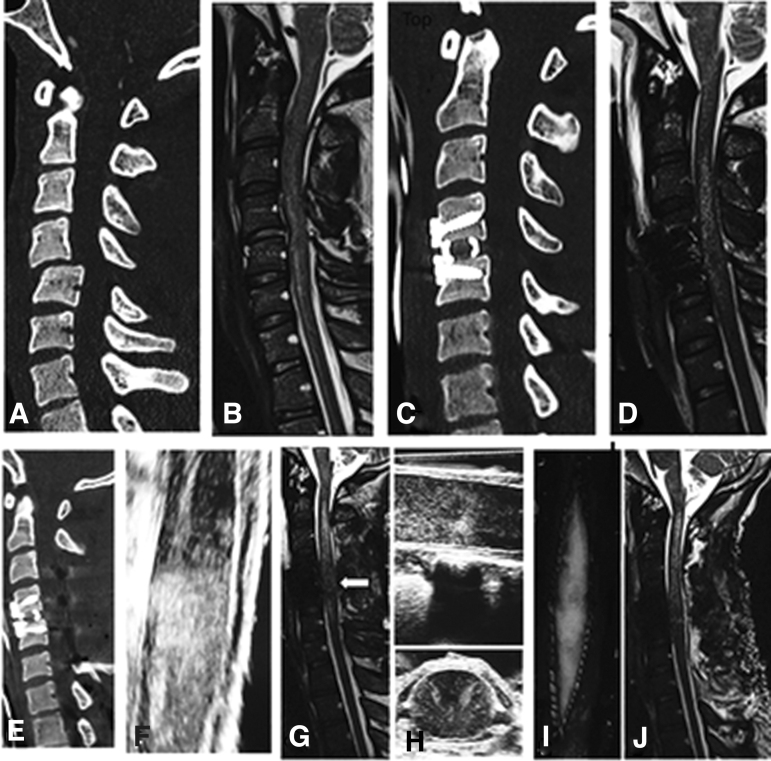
Midsagittal computed tomography and magnetic resonance imaging (MRI) views (plates A and B) from a 20-year-old male patient with C4/5 unilateral locked facets and quadriplegia during a friendly game of wrestling. Plate A indicates translation rotation injury between C4 and C5 (AOSpine injury type C^28^) vertebral bodies 6 h after trauma. Nine hours after traction reduction, the MRI indicated lack of cerebrospinal fluid (CSF) interface in front and in the back of the spinal cord from C2–C6 and an intramedullary lesion length of 73.1 mm. (plate B). After anterior cervical discectomy and fusion, MRI (plate D) indicated continued absence of CSF in front and in the back of the spinal cord. The patient had a second stage surgery that was laminectomy. Real-time intraoperative ultrasonography after laminectomy revealed spinal cord swelling with a thin interface in front of the spinal cord; however, the surgeon was not convinced expansive duraplasty was necessary (plates E and F). Post-operative MRI (plate G) revealed absence of CSF around the spinal cord at C4/5 skeletal segments. Although intraspinal pressure monitoring was not available at the time, the patient was re-explored for expansion duraplasty (plates H, I, and J), which re-established CSF interface around the spinal cord.
